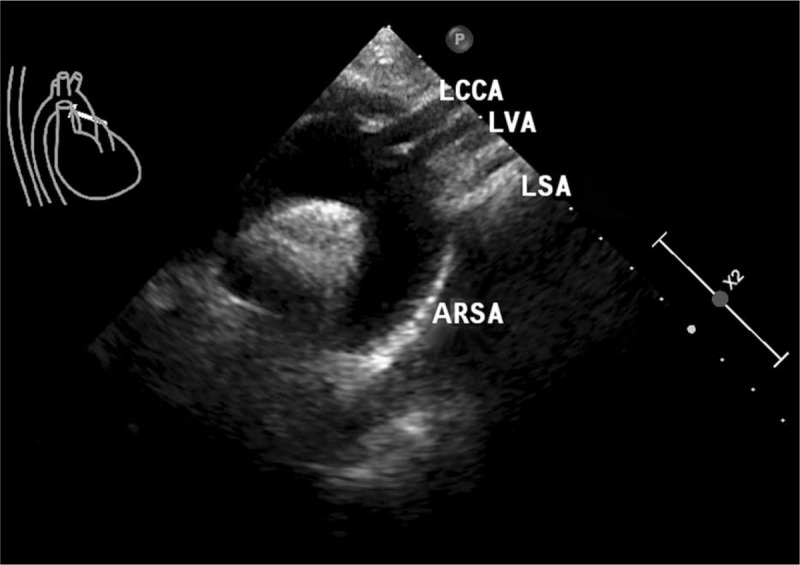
Echocardiography showing the variations of AA branches.
